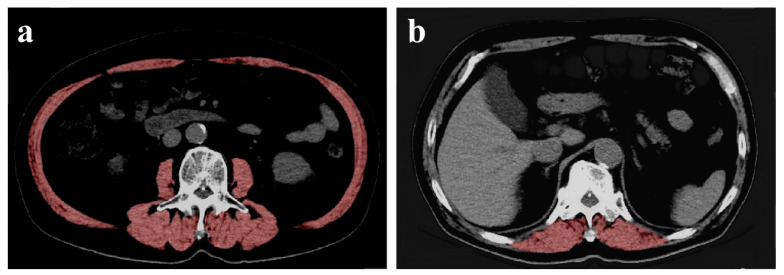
Example of L3 and Th12 delineation using ImageJ. (a,b) show the cross-sectional computed tomography (CT) images of L3 and Th12, respectively. The cross-sectional area of the muscle in the red area is measured.
